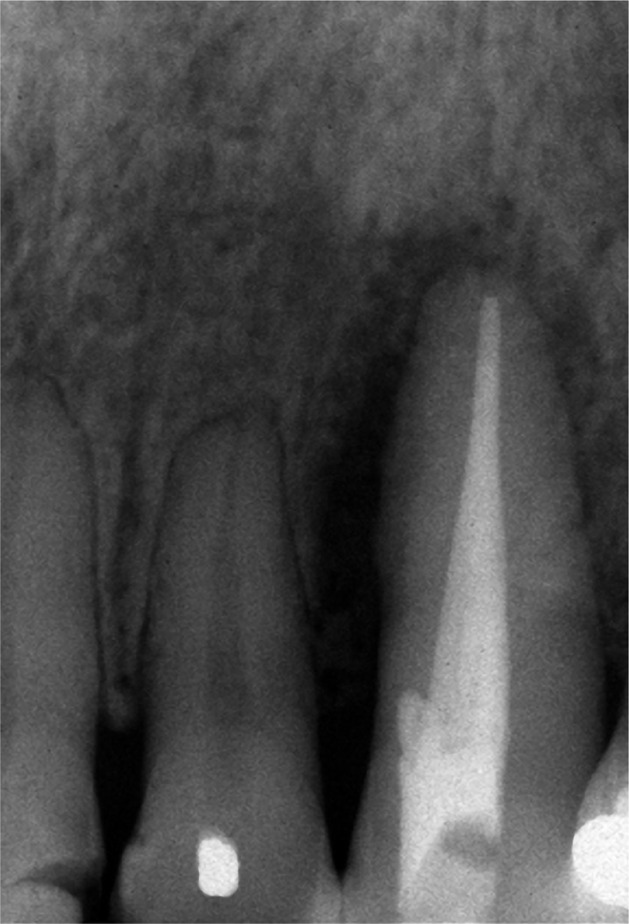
Four months after the endodontic treatment, the radiograph shows a slight decrease in lateral and periapical radiolucency.
